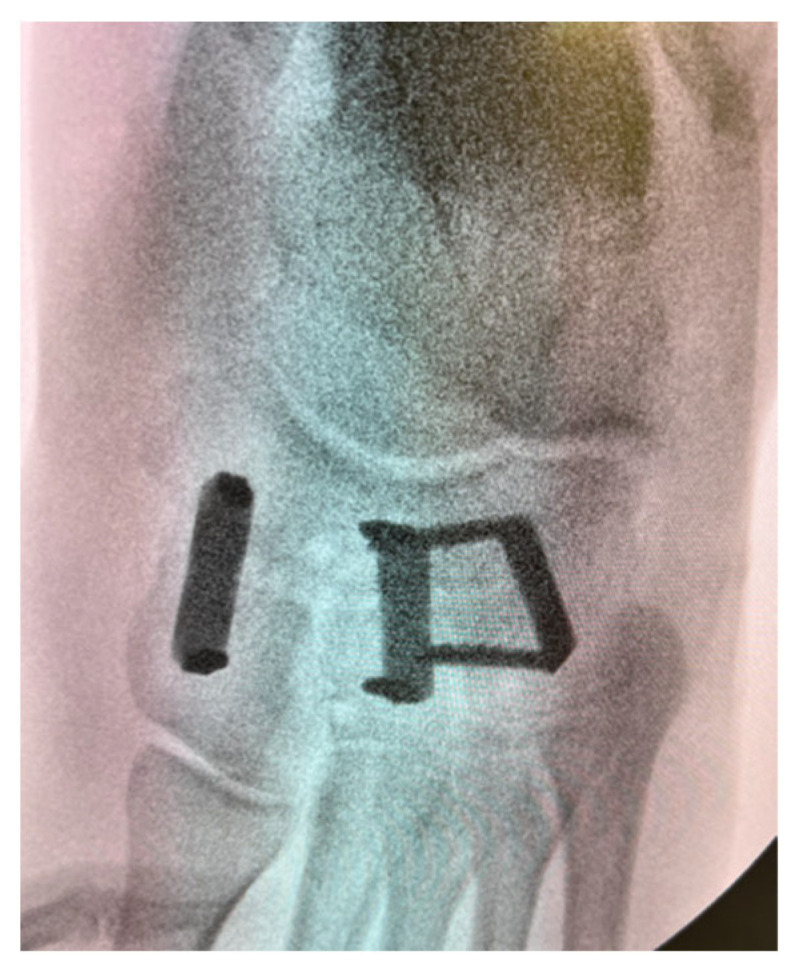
Intra-operative anteroposterior radiograph depicting a naviculocuneiform joint fusion with CCI fixation.
